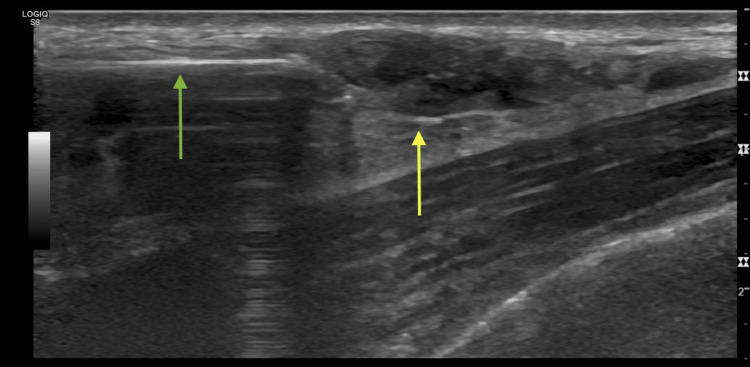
Ultrasound biopsy Ultrasound biopsy findings stated that "The mass in the left breast at 11 o'clock, 11 cm from the nipple, was successful. Pathology indicates malignant invasive adenoid cystic carcinoma (ADC). Pathology results are concordant with imaging findings." Green arrow: biopsy needle Yellow arrow: mass
